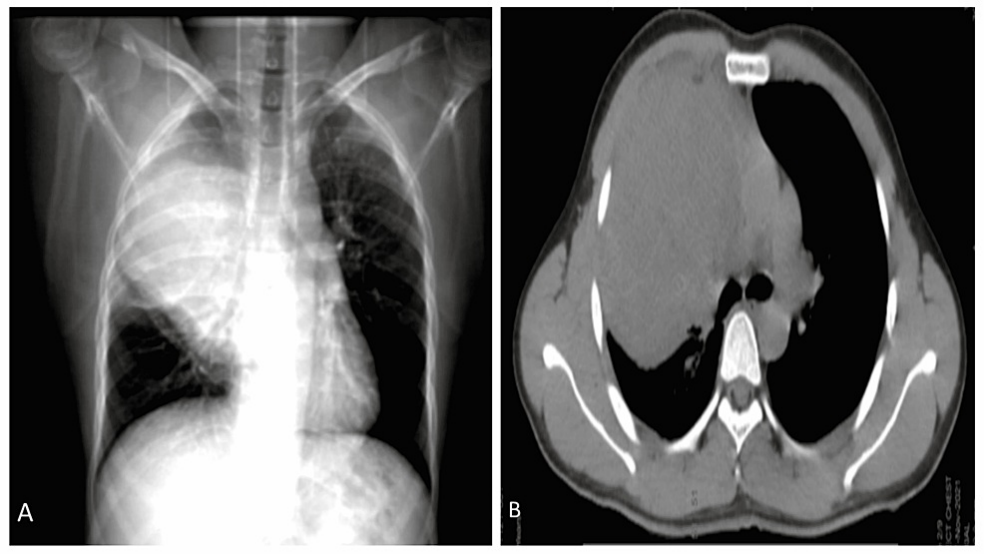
Radiological images (A) Chest X-ray shows large, homogenous soft tissue density mass lesion occupying the right upper lobe region. (B) CECT scan shows a large mass in the right lung region which reveals rib destruction and extends across the chest wall. The mass shows peripheral necrotic areas.
